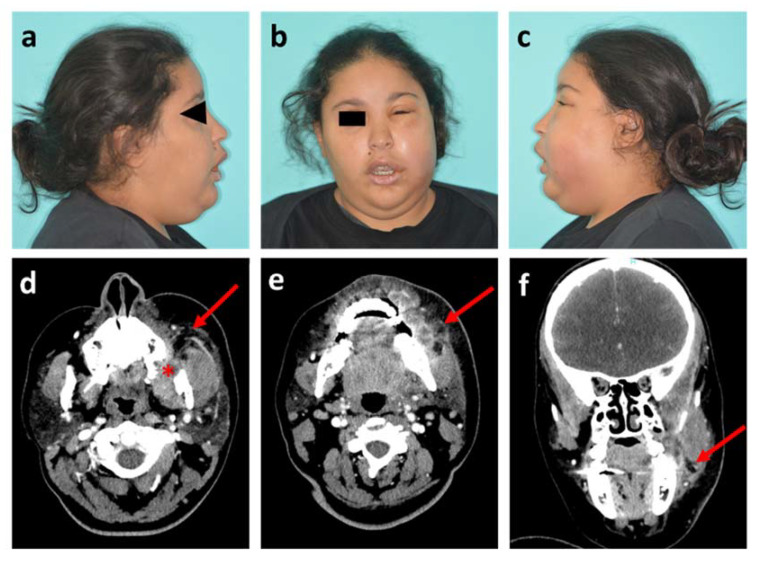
Photographs and head and neck CT scan of patient 1. (a–c) Visible images of healthy right profile, front view and affected left profile, respectively; (d,e) Axial plane of CT scan in soft tissue window, at maxillary level with the socket of the tooth #28 (red star) and at mandibular level, respectively; (f) Sagittal plane of CT scan. Red arrows show the infectious collection.
